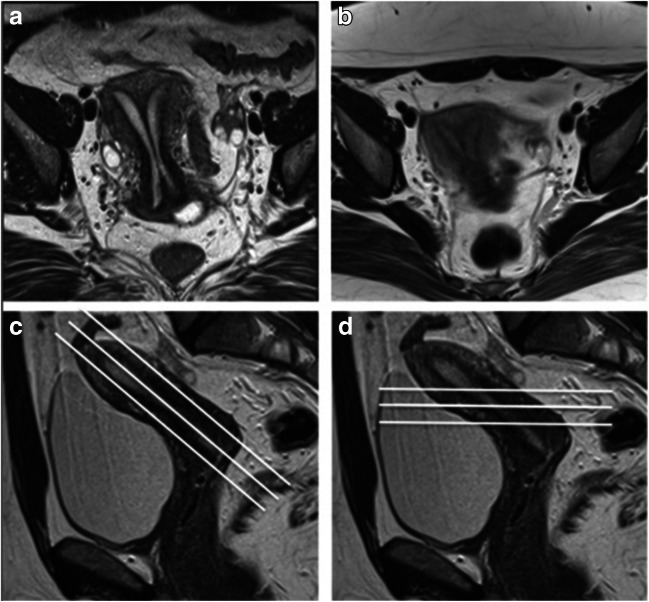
Importance of a dedicated MRI protocol. The same patient and the same MRI scanner but two examinations a few days apart. The patient was recalled to perform vaginal filling with US gel, for better characterisation of a vaginal septum. Axial T2W TSE images (a, b). The superior quality of image a is obvious, due to slice thickness (3 mm versus 5 mm), small FOV and prescribing the appropriate orientation. Image a depicts properly the uterine fundus and septum, important features for characterisation and classification of this uterine malformation (complete septate uterus). c, d Planning the acquisition from the sagittal sequence: axial oblique acquisition oriented by uterus long axis (c) yielding to a true coronal acquisition of the uterus shown in a versus an axial strict acquisition of the pelvis (d) yielding image b
